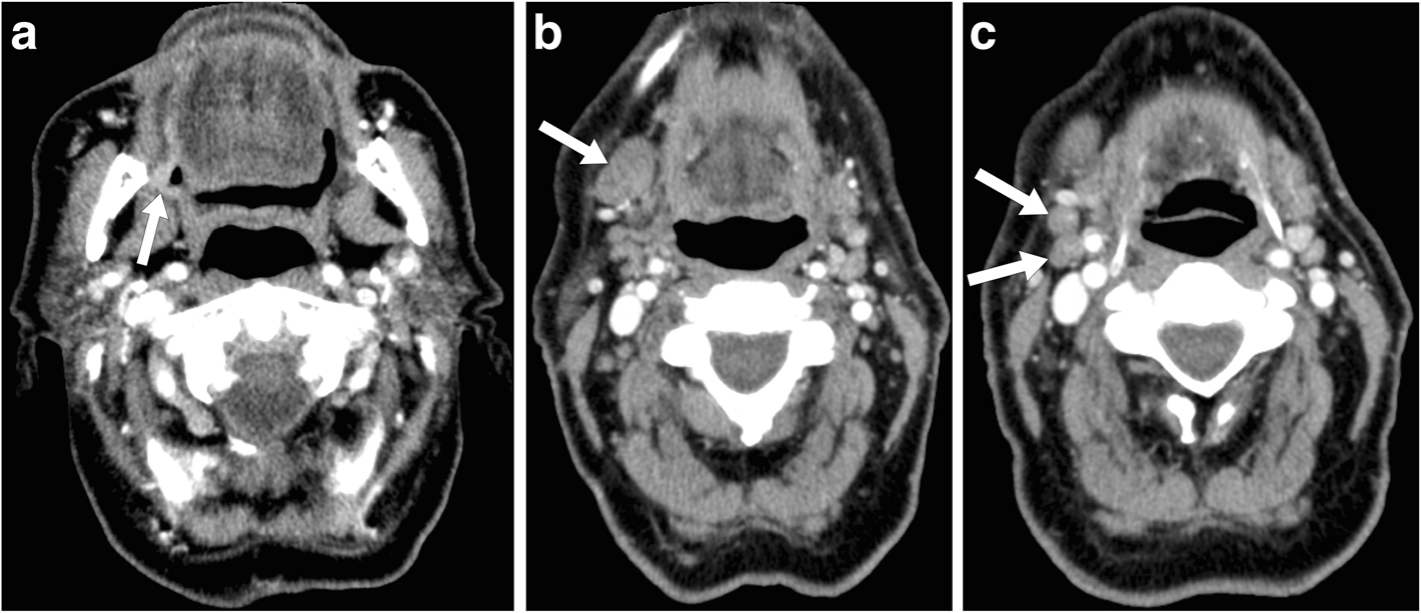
Axial contrast enhanced CT images of the neck in an 81 year old male (Patient Z in Table 3); a fine needle aspiration biopsy of a clinically enlarged right submandibular lymph node had shown squamous cell carcinoma. (a) A plaque-like (2.5 cm in diameter × 0.5 cm in maximum thickness) enhancing mucosal lesion involving the right retromolar trigone (arrow) was not mentioned in the original radiology report but was identified as the primary tumor in the second opinion report. (b) An enlarged (2.3 cm × 1.5 cm) right level IB lymph node (arrow) was interpreted as metastatic in both the original and second opinion reports. (c) Two sub-centimeter but rounded and asymmetrically prominent right level IIA lymph nodes (arrows) were not mentioned in the original report but were interpreted as metastatic in the second opinion report. Staging was TxN1 based on the original report and T2N2b (Oral Cavity) based on the second opinion report, and the recommended management was ‘don’t know’ based on the original report (because the primary tumor location and T-category were unclear) and ‘surgery’ based on the second opinion report. Surgery was subsequently performed and the final pathologic staging was T2N2b (Oral Cavity).
